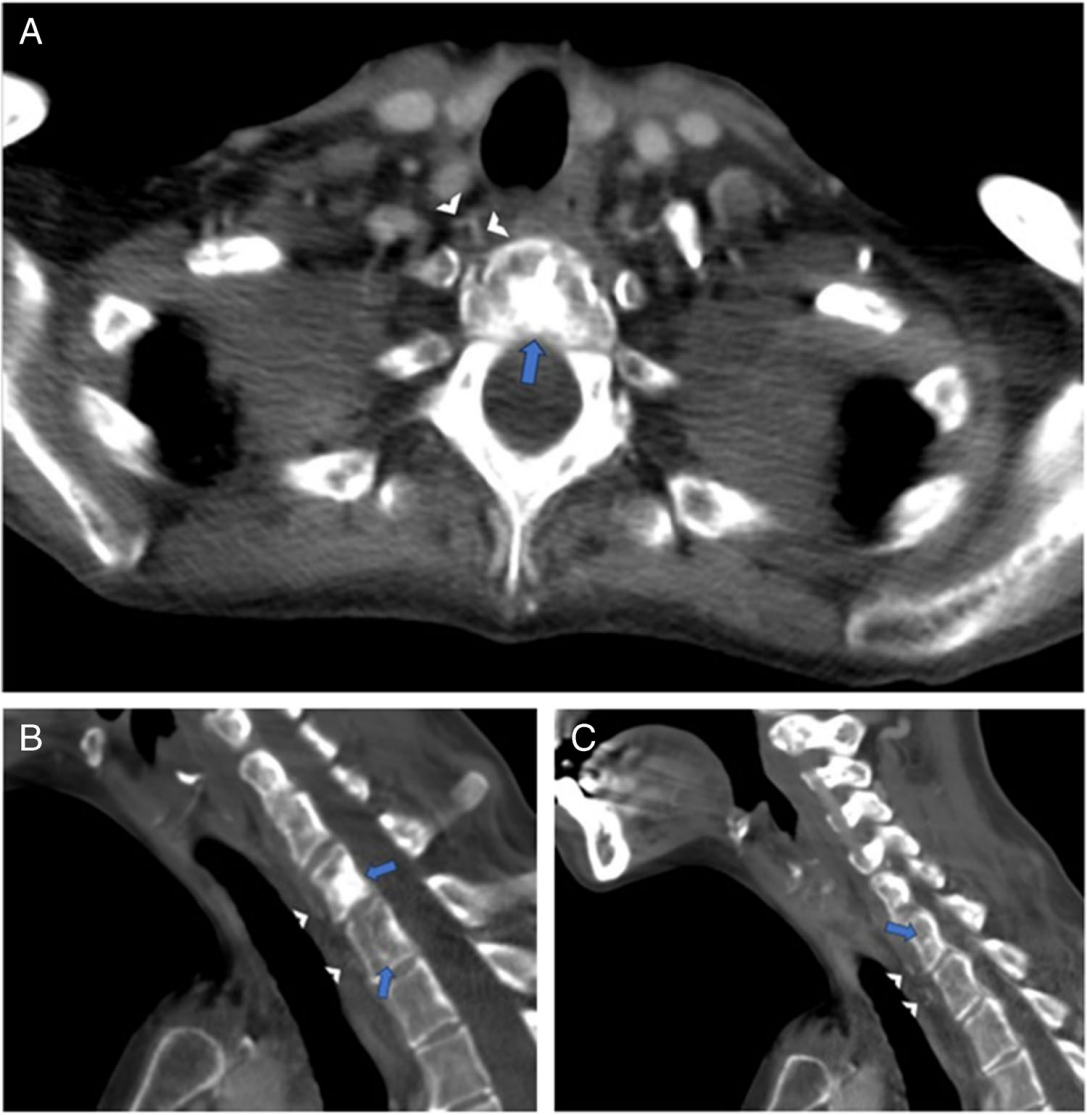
Axial (A) and sagittal (B, C) images from contrast-enhanced neck CT (the same study presented in Fig. 1) demonstrate tiny anterior paravertebral venous collaterals (arrowheads) adjacent to the hyperdense vertebral body foci (arrows)
